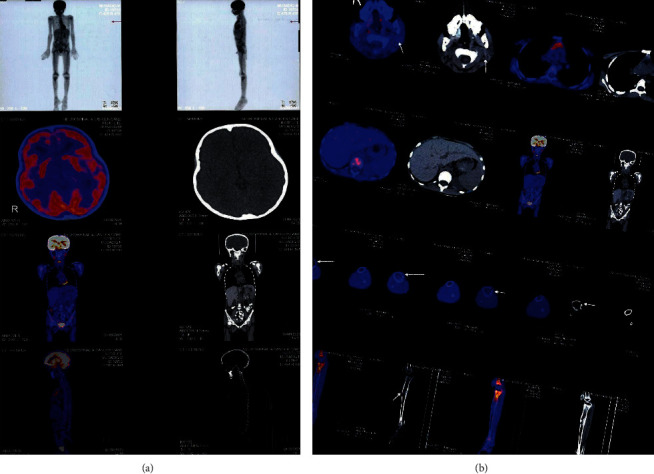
(a) PET-CT posttreatment and (b) PET-CT posttreatment.
